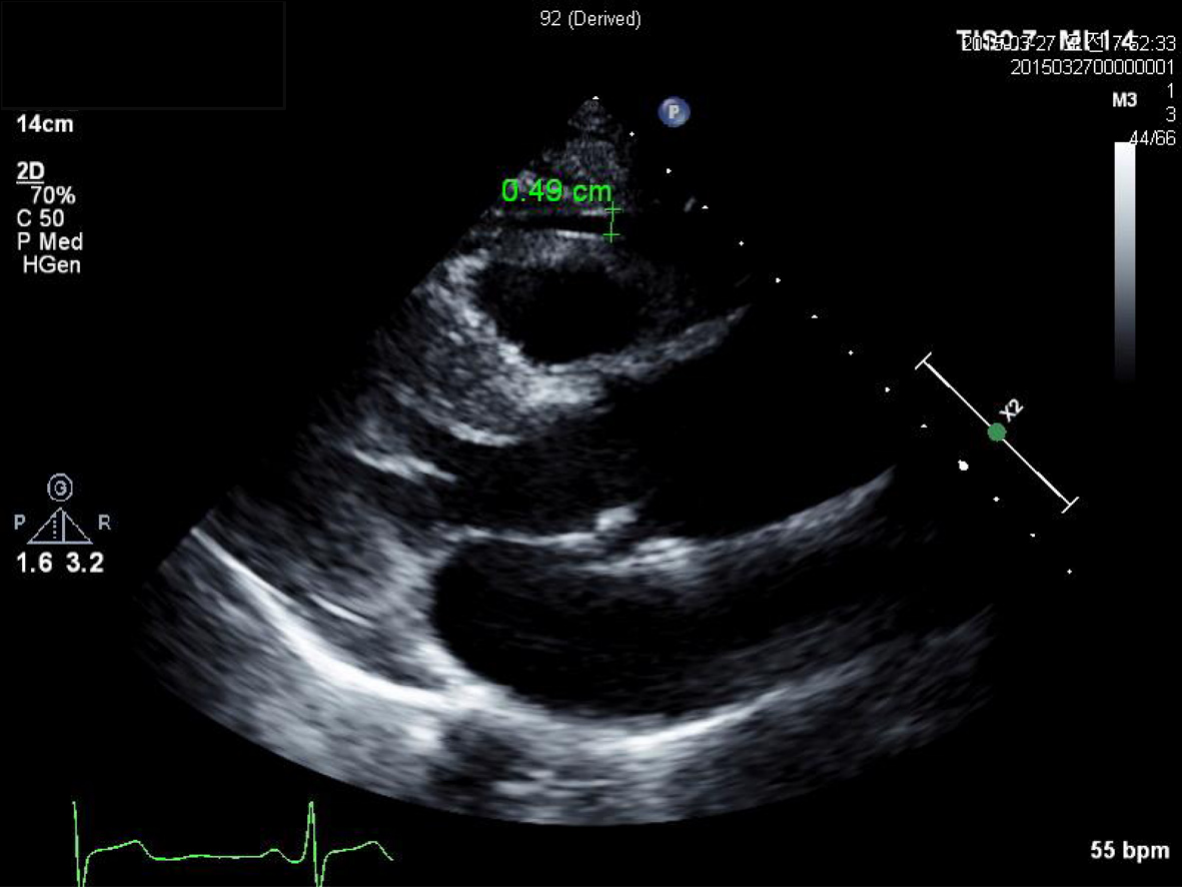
Echocardiographic assessments of EFT, defined as the echo-free space between the outer wall of the myocardium and the visceral layer of the pericardium, were measured perpendicularly from the free wall of the right ventricle at end-systole in the parasternal long axis view
